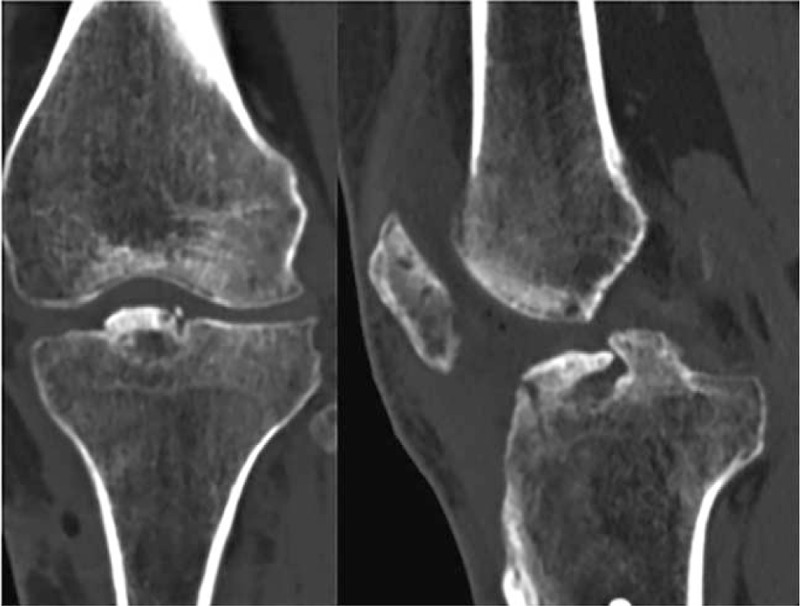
Postoperative CT scan showing satisfactory fracture reduction and healing in the case of ununited tibial eminence fracture. CT =computed tomography.
